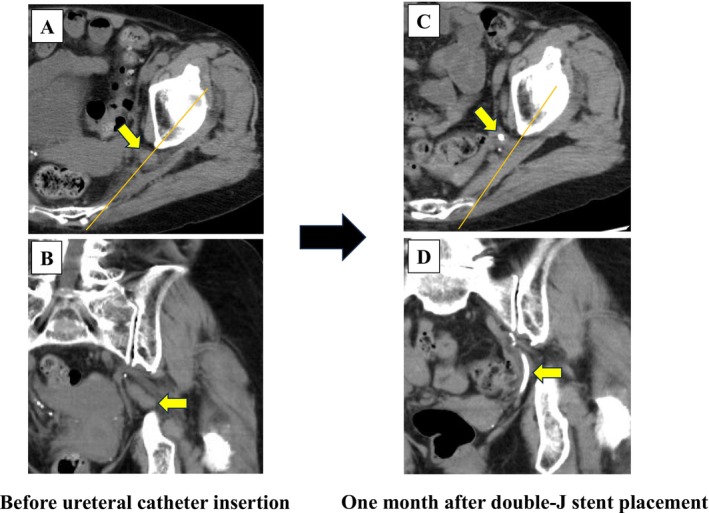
Serial CT images before and after resolution of USH. (A, B) CT images of left USH before ureteral catheter placement (top: Axial view; bottom: Coronal view). (C, D) CT images at 1 month after double‐J stent replacement (top: Axial view; bottom: Coronal view). The orange lines indicate the line connecting the ischium and sacrum. The yellow arrows indicate the left ureter.
